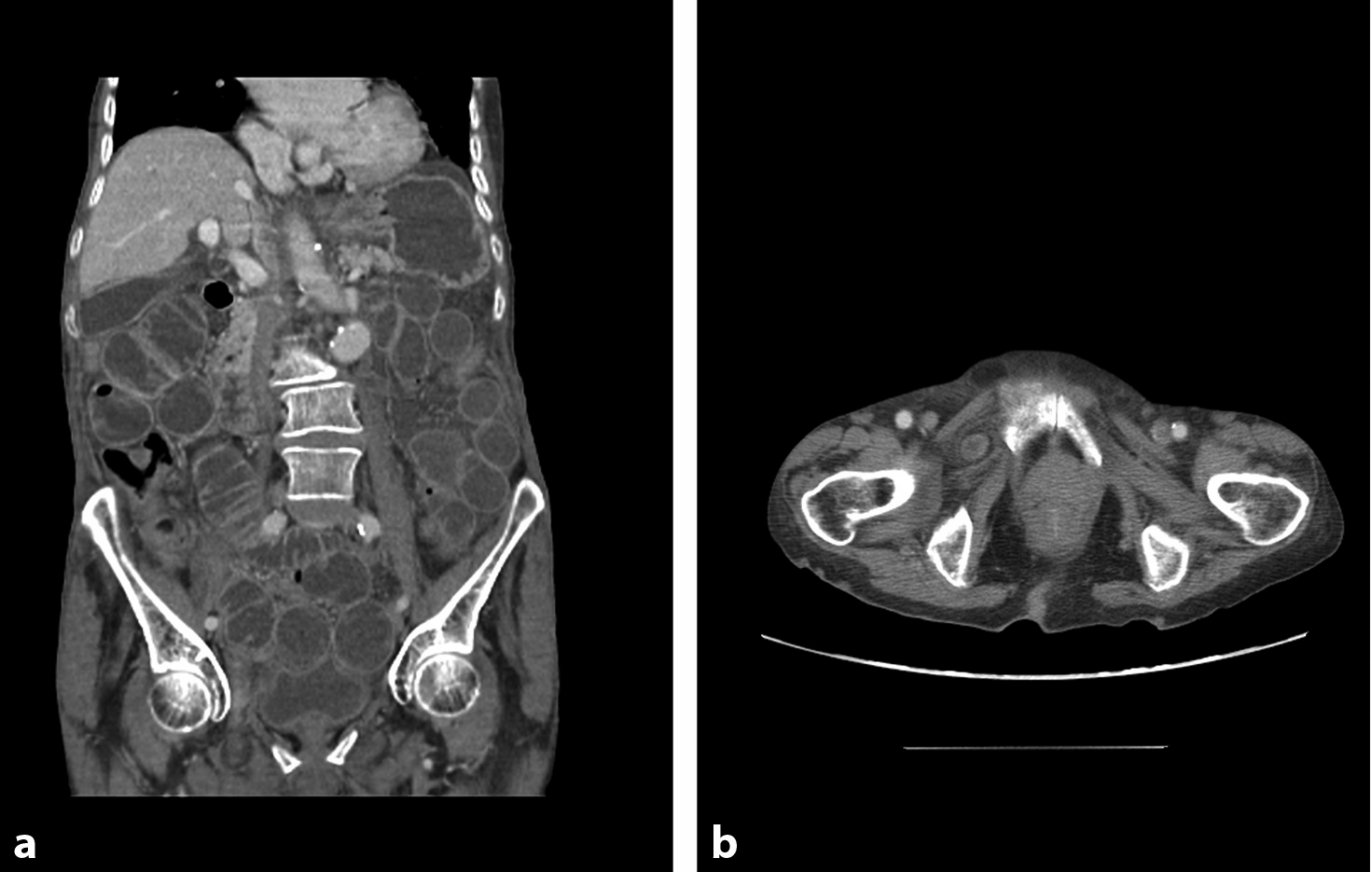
(a) Coronal CT scan of the abdomen and pelvis showing incarceration of a portion of the ileum in a right obturator hernia with transition point. (b) Axial CT scan of the pelvis showing a portion of the ileum in a right obturator hernia.
